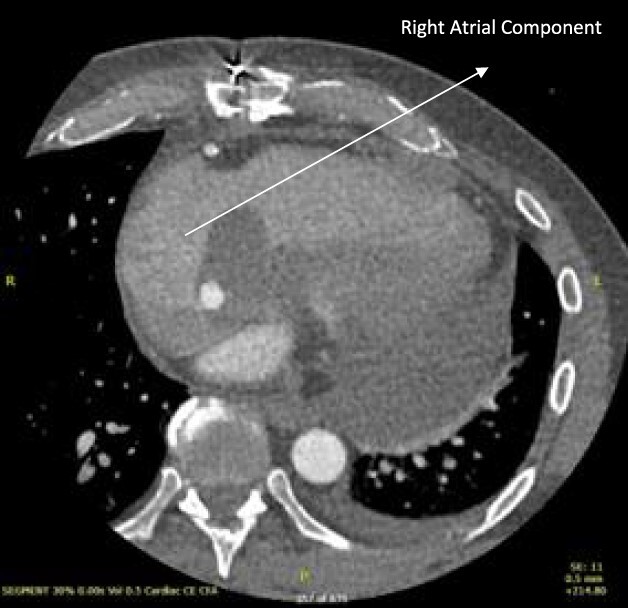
Post-operative CT scan showing further reduction of RA bulk following chemotherapy.
